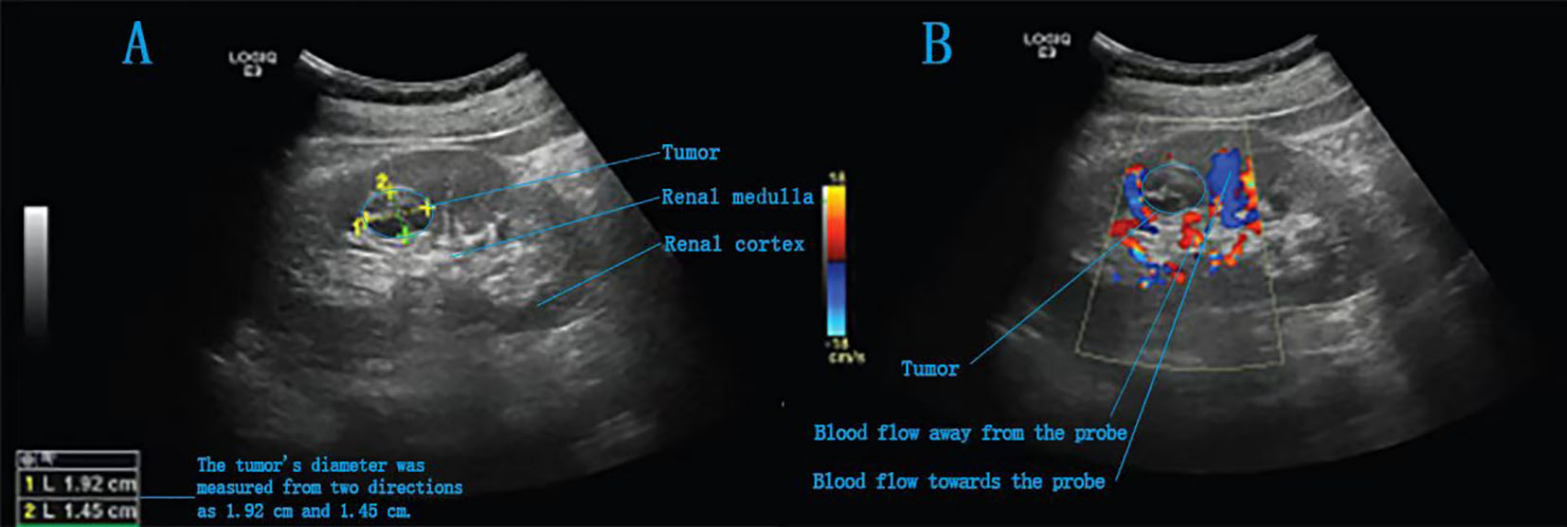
Intraoperative ultrasound images of a completely endogenous renal tumour. (A) A slightly hyperechoic area is detected in the right kidney, with heterogeneous internal echotexture. (B) Intraoperative Doppler ultrasound image.
